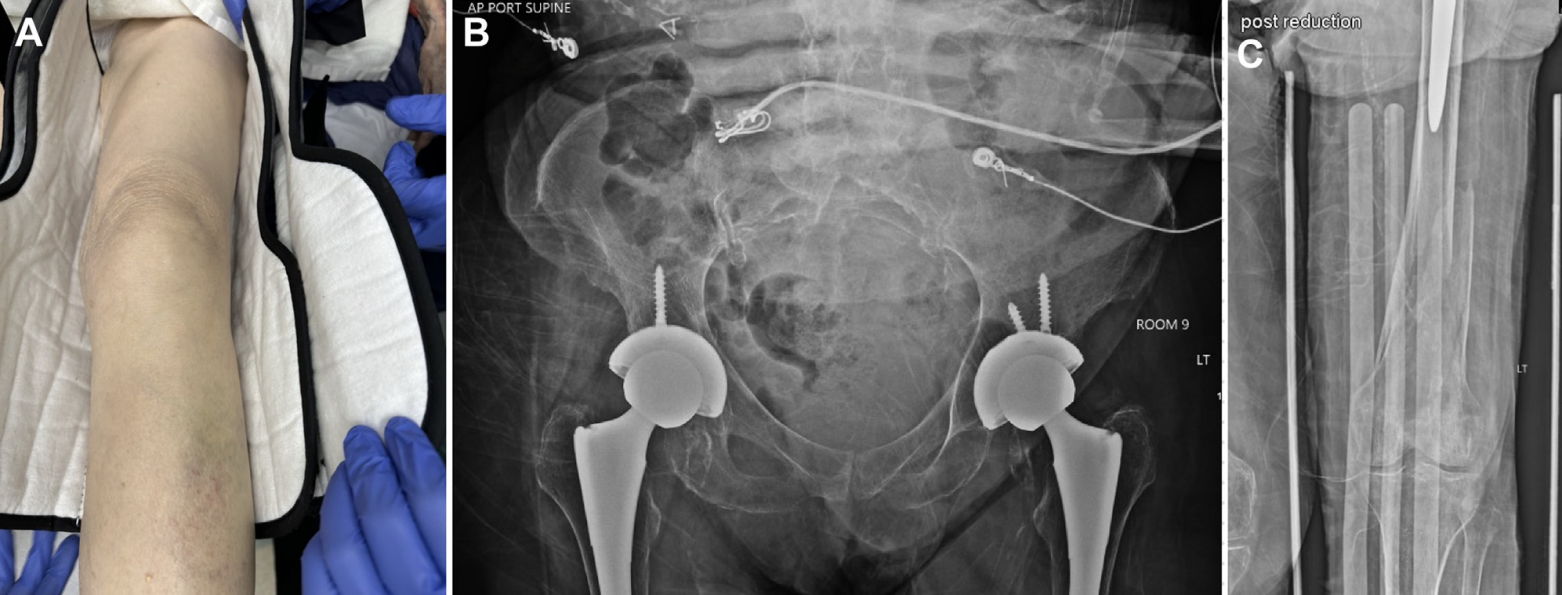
(A) The previously deformed and rotated left leg is now positioned in anatomic alignment. Single-view, postreduction, anteroposterior radiographs of the pelvis (B) and femur (C) showed bilateral total hip arthroplasty with acetabular screws but no loosening, fracture, or dislocation and the femoral shaft fracture.
